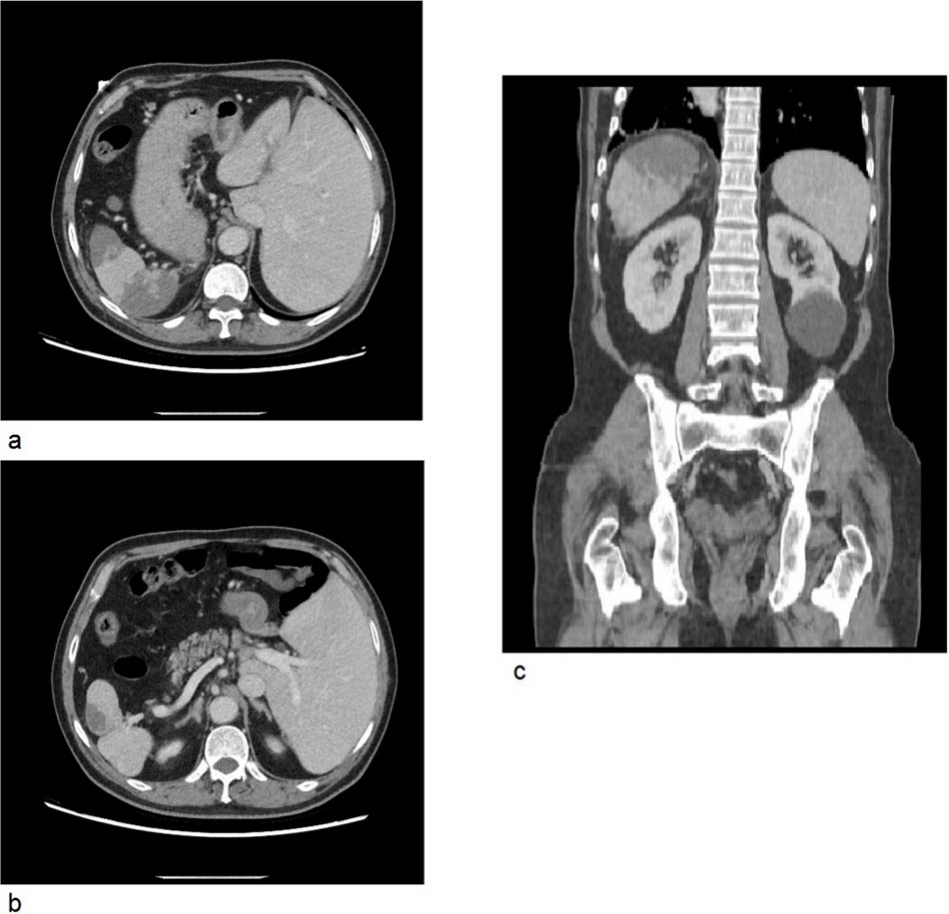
(a) CT axial cross section showing the liver on the left side of the abdomen and the spleen on right side with multiple splenic infarcts. (b) CT axial cross section showing patent splenic vein. (c) CT coronal cross section showing splenic infarcts as well as a left kidney cyst.
